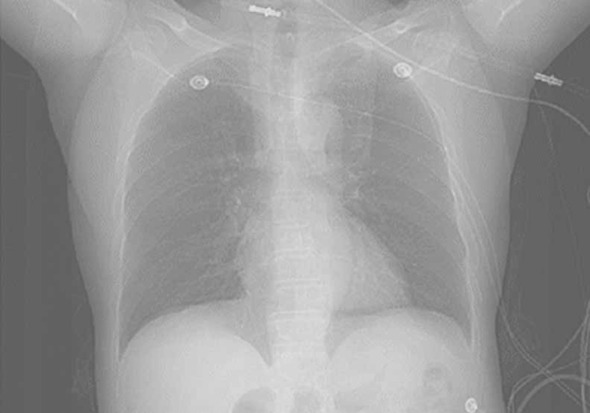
Chest X-ray shows a slightly rough shadow at the hilum of the lung.
